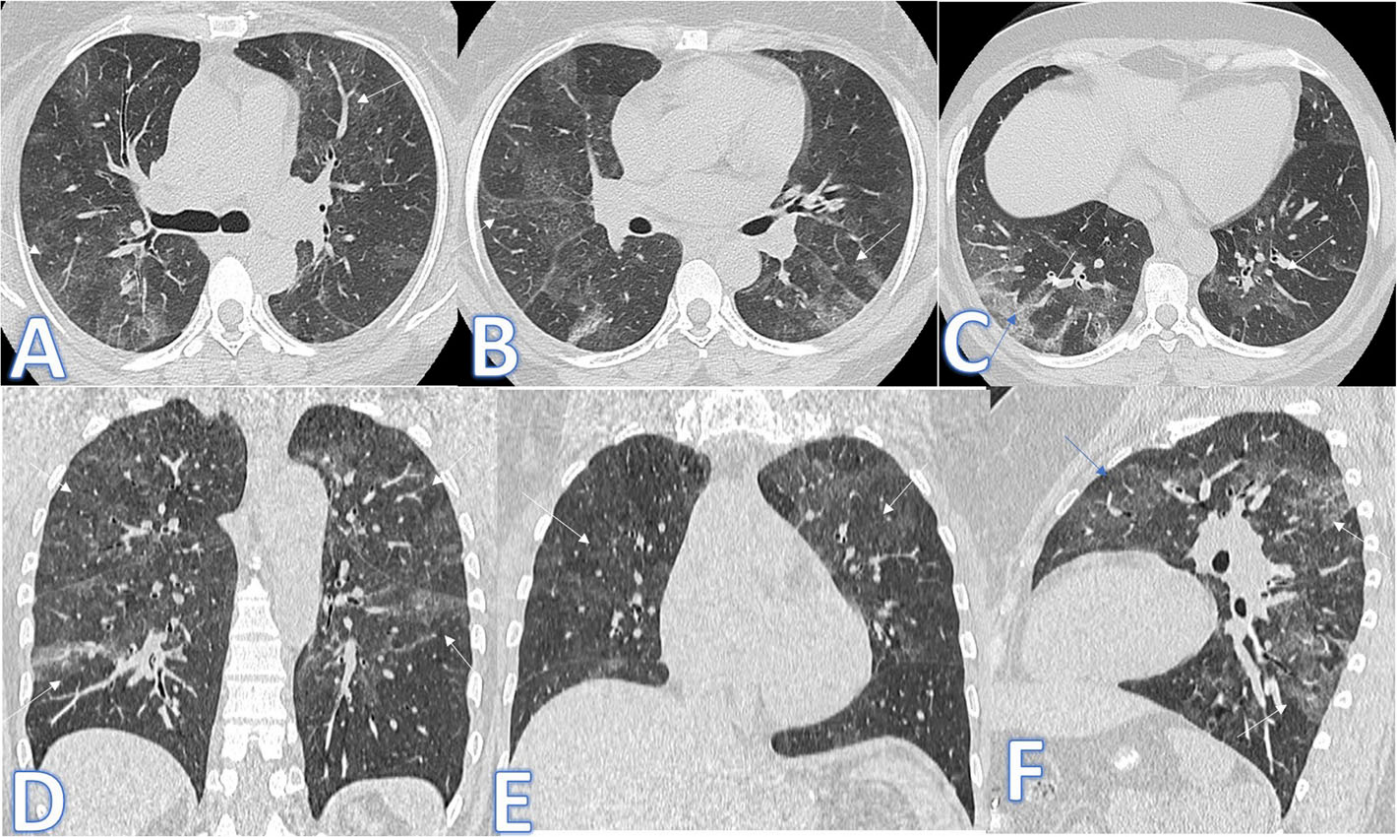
A 45-year-old male patient presented with cough and dyspnea. CT showed bilateral patches of GGO in both lobes (white arrows) with crazy paving seen in both lower lobes (blue arrow); the diagnosis was RB-ILD proven by biopsy
